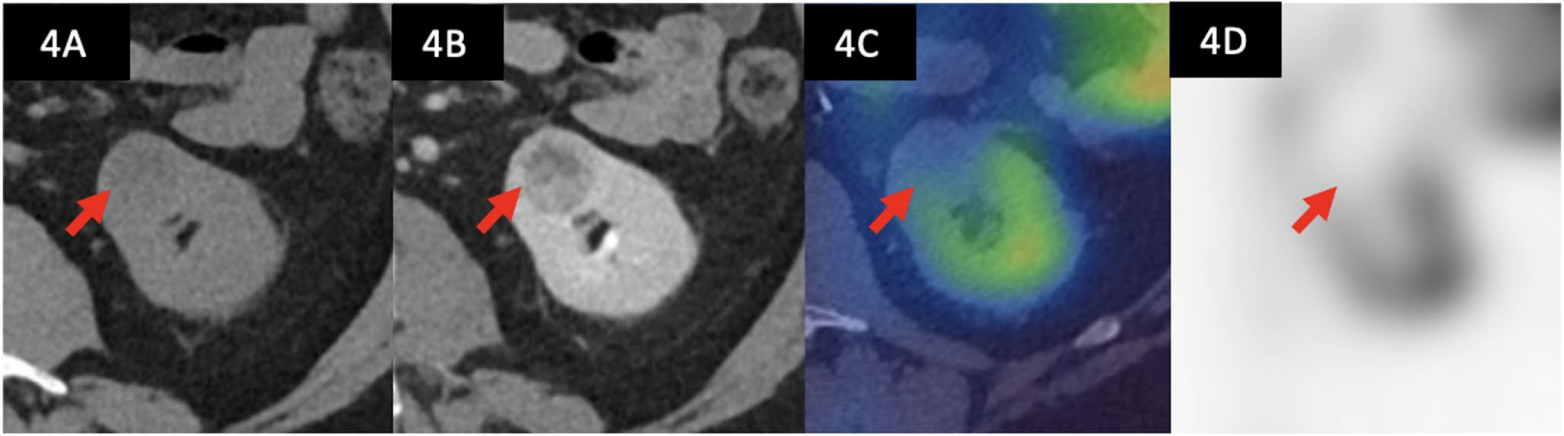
Diagnostic imaging of a 24 mm left anterior tumour indicated by the red arrow, diagnosed on biopsy as a clear cell renal cell carcinoma. This tumour was interpreted as photopaenic relative to the normal parenchyma of the ipsilateral kidney A) axial non-contrast CT of the left kidney B) corresponding contrast-enhanced CT demonstrating a solid, enhancing, heterogenous tumour (C) axial [^99m^Tc]Tc-sestamibi SPECT-CT images shows no radiotracer uptake in the region of the tumour (D) corresponding axial SPECT image shows no [^99m^Tc]Tc-sestamibi uptake [Computed tomography = CT, Single photon emission computed tomography = SPECT]
